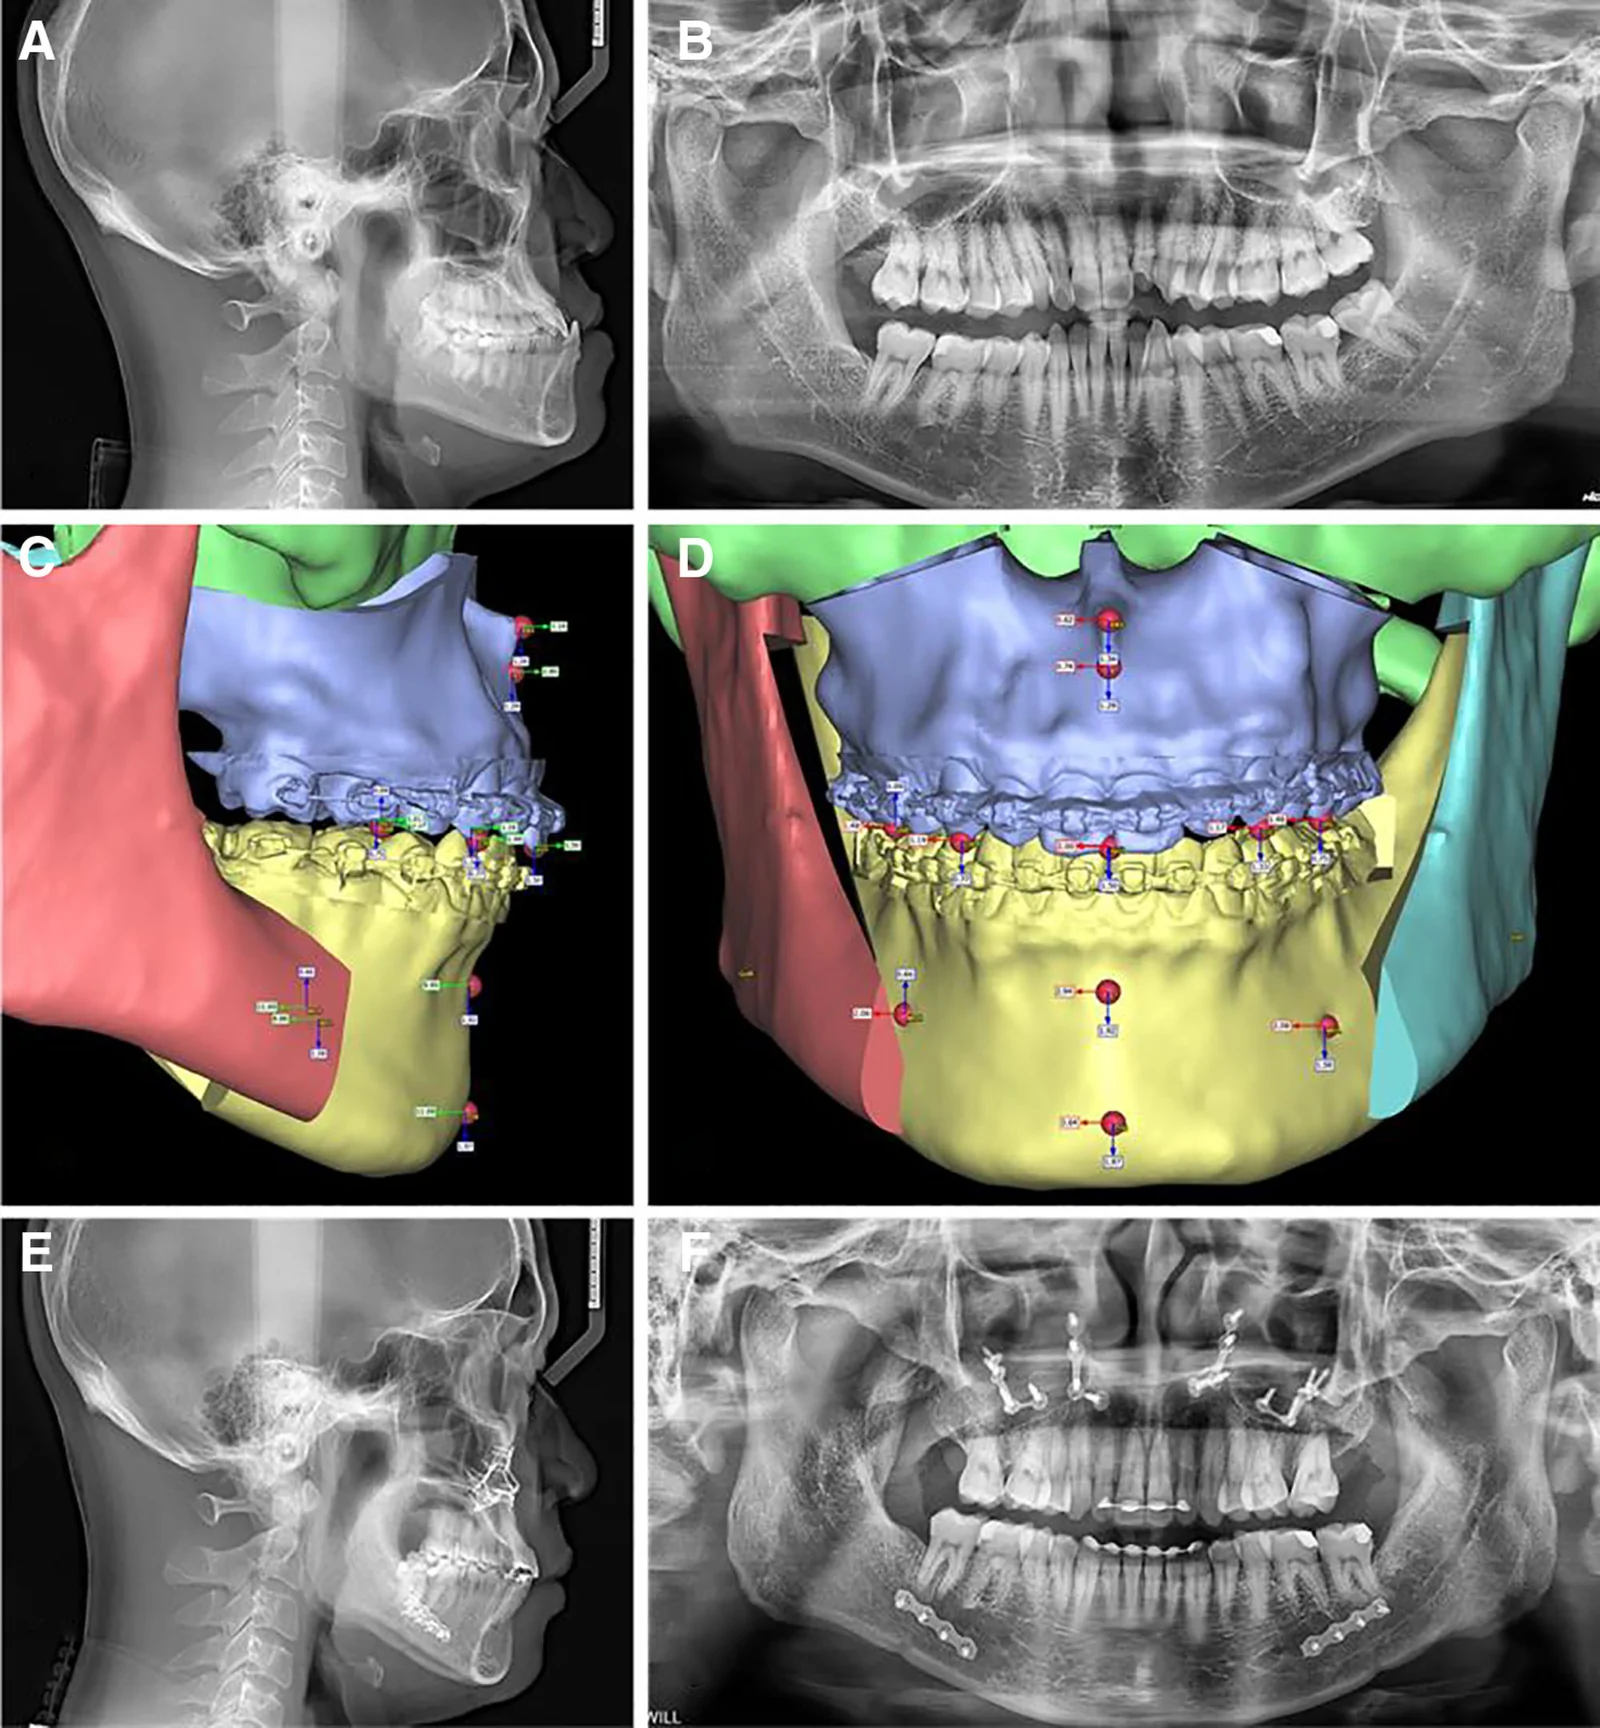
Case 1, radiographs. (A, B) Pretreatment. (C, D) Surgical plan. (E, F) Posttreatment.
